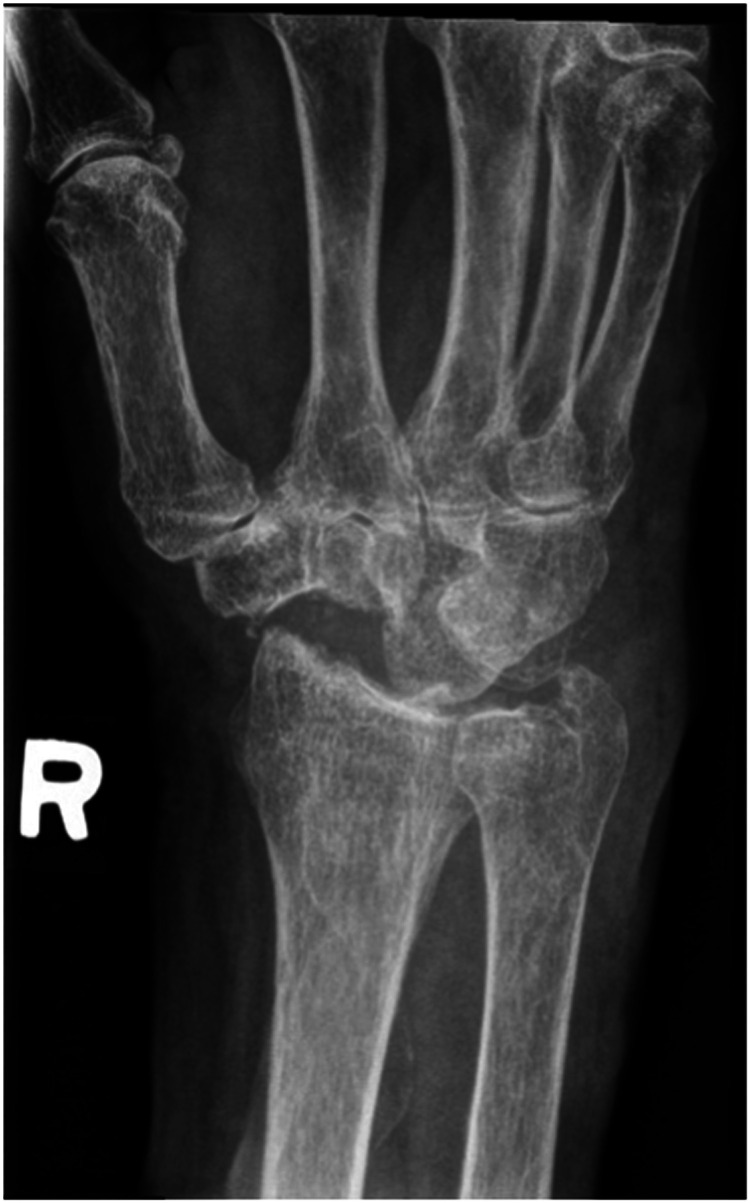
Postoperative right hand plain film oblique view x-ray.
